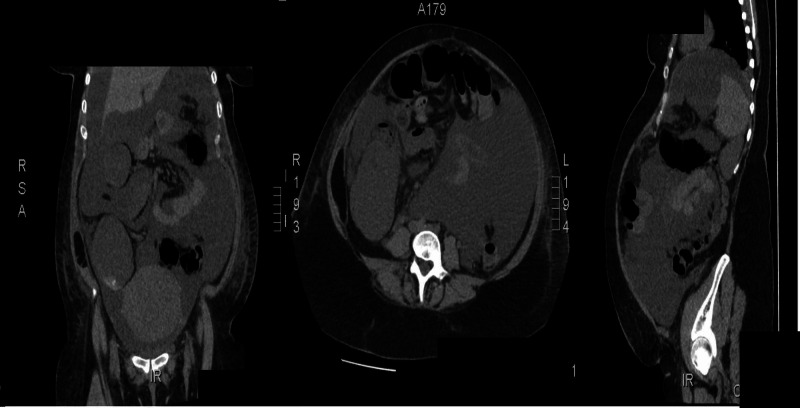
Non-contrast CT scan with large amount of intraperitoneal fluid accumulations
